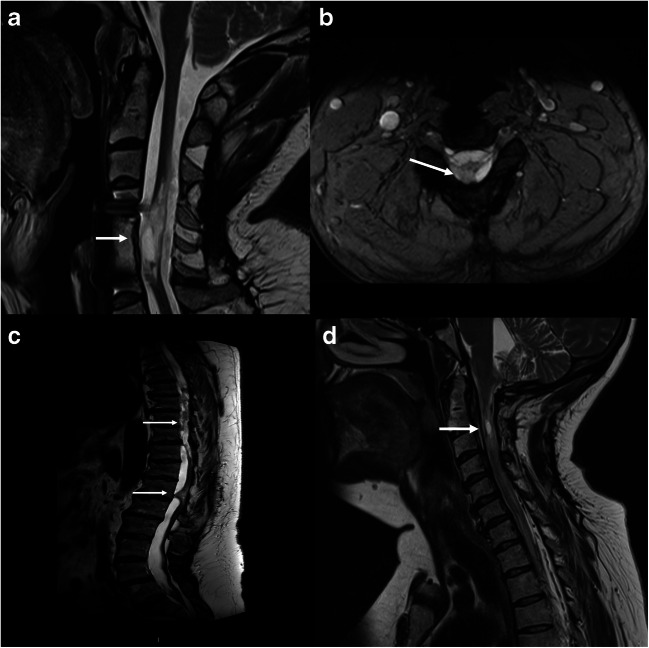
Preoperative magnetic resonance imaging (MRI) of three patients included in the present cohort. a Evidence of myelomalacia with spinal cord tethering over 10 years following a traumatic spinal injury, sagittal image (arrow). b Spinal cord tethering at C5, axial image. The patient was treated surgically with laminectomy, untethering of the spinal cord and duraplasty (arrow). c Patient sustaining an intradural hemorrhage from a routine spinal tap that resulted in neurological deficits and emergency surgical evacuation. At 12 months following the hemorrhage, a gradual yet marked neurological deterioration of lower limb sensory and motor function was observed which was treated by laminectomy, spinal cord untethering, and duraplasty. Note the marked adhesions and deformation of the spinal cord (arrows). d Intradural scarring, an intramedullary cyst (arrow), and spinal cord signal changes were found in a patient with previous meningitis and gradual neurological deterioration who was surgically treated by intradural exploration and release of scar tissue. This patient had the highest CSF GFAP levels in the current cohort
